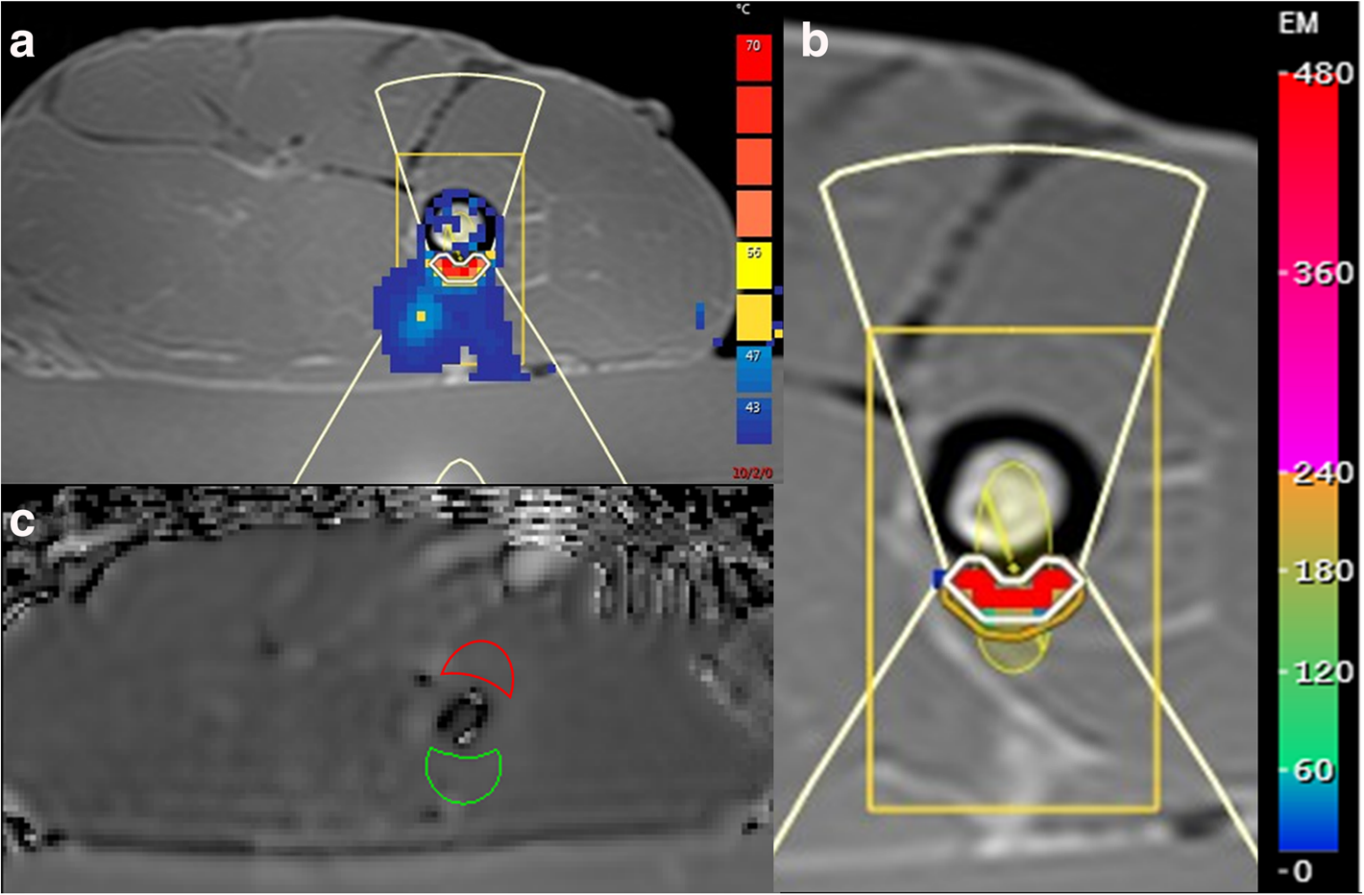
a T1-weighted image showing a lamb leg sample placed on a gelpad for sonication, with the heated region indicated by a colour overlay of the temperature map derived from PRFS (red pixels > ~60 °C). The sonicated cell (yellow ellipsoid) is shown magnified for greater clarity in (b), with the heated region shown in units of thermal dose (red pixels > ~400 equivalent minutes (EM)). The white line indicates the 240 EM at 43 °C thermal dose contour. ROI positions representing the heated region on the relevant slice of the ADC map (green outline), and a control region placed distally (red outline) are shown in (c)
